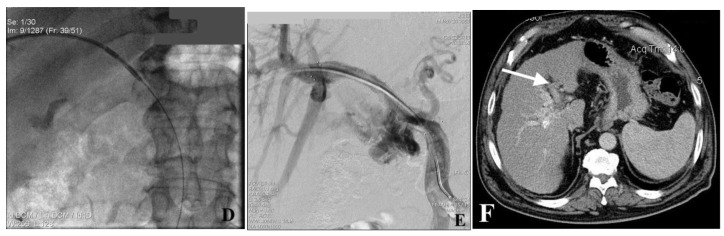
Images from a 60-year-old male patient diagnosed with HCC complicated with PVTT. (A) Axial CT image shows that the HCC lesion completely occupied segments 2 and 3, the left PV is obliterated by the tumor thrombus (white arrow), while the right PV remains patent (red arrow). (B) VesOpen procedure images in succession—the portography “above” the thrombus (PAT) shows the right PV patent branches. (C) VesOpen procedure images in succession—the portography “below” the thrombus (PBT) shows a dilated SMV interrupted by the tumor thrombus at the level of PV confluence; the main PV is not opacified. (D) Tumor thrombus processing by bipolar endoluminal VesOpen RF device. (E) Adequate recanalization of the PV-obstructed segment is seen after stent implantation. (F) Follow-up CT image (5.5 months after the procedure). The patient did not receive any other treatment. The left lobe tumor shrinkage and the not-treated left PV recanalization (white arrow) are clearly documented—abscopal effect of PV tumor thrombus RF processing.
